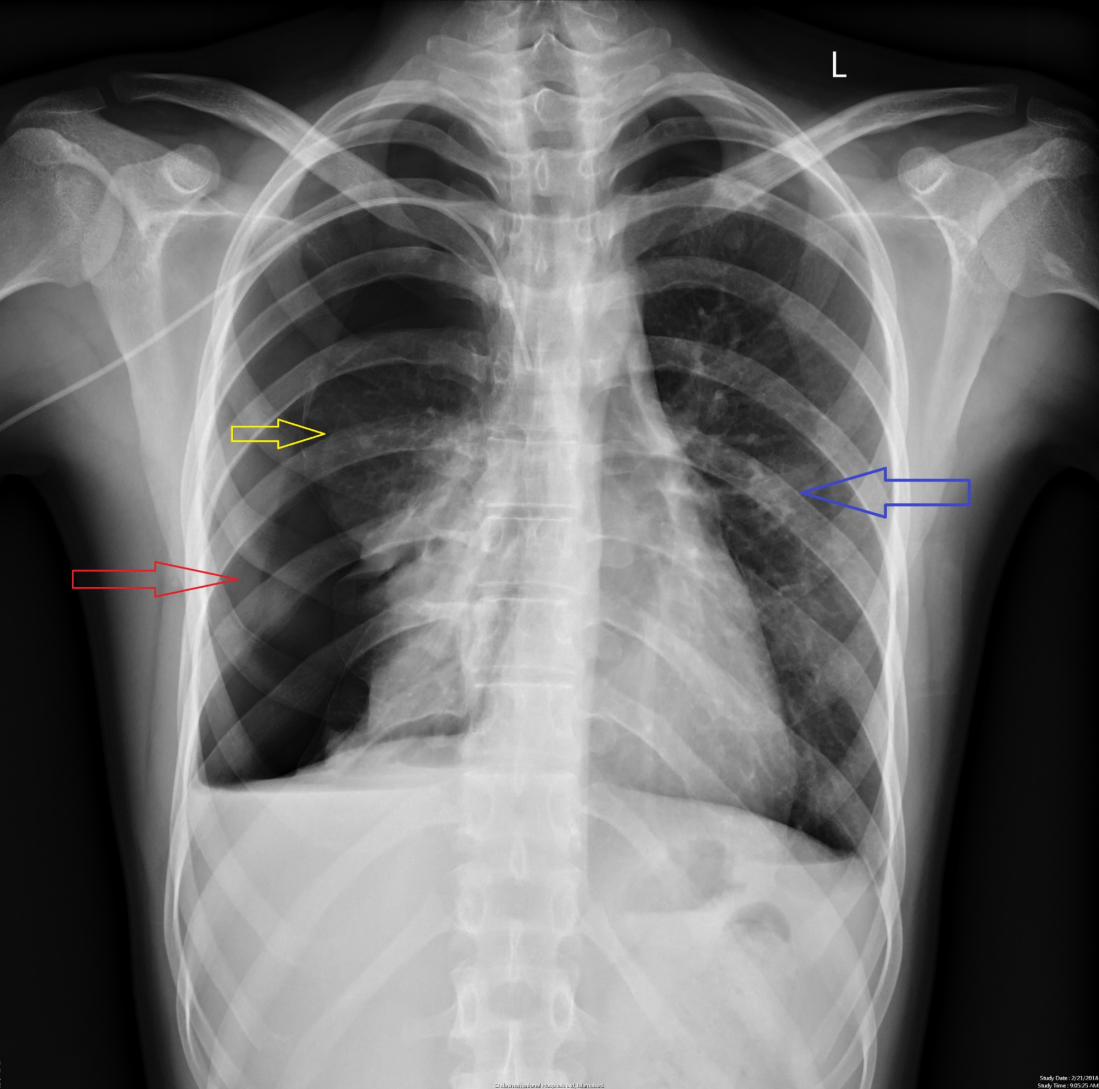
Chest X-ray showing recurrent pneumothorax on the right side. Red arrow points toward an extensive area of radiolucency on the right side signifying right sided pneumothorax. Yellow arrow points toward medially displaced lung parenchyma on the right side. Blue arrow points toward normal lung on the left side.
